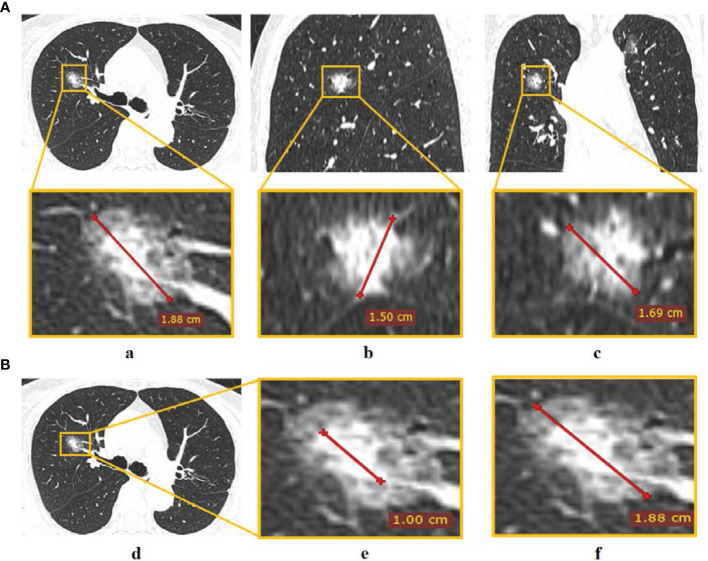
The measurement of CT radiological features. (A) Tumor diameter measured in (a) transverse, (b) sagittal, and (c) coronal planes, and the largest diameter in the three planes was defined as MTD; (B) C/T ratio measurement of (d) tumor *via* (e) maximum consolidation diameter divided by (f) maximum tumor diameter in the transverse plane.
